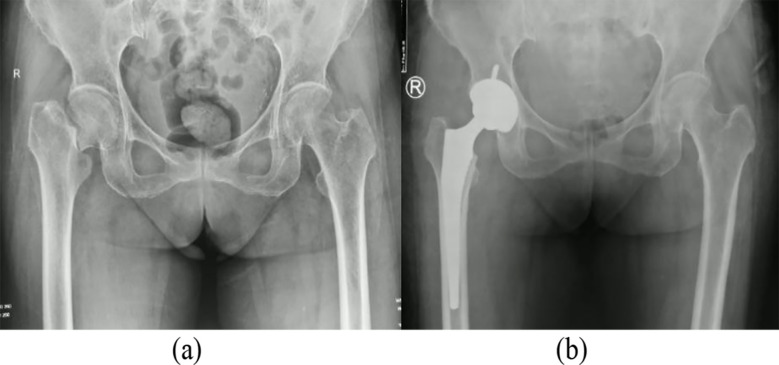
Preoperative and postoperative X-ray images of THA. *Note:* Female, 68 years old, underwent THA for a right femoral neck fracture. (a) Preoperative X-ray; (b) Postoperative X-ray.
